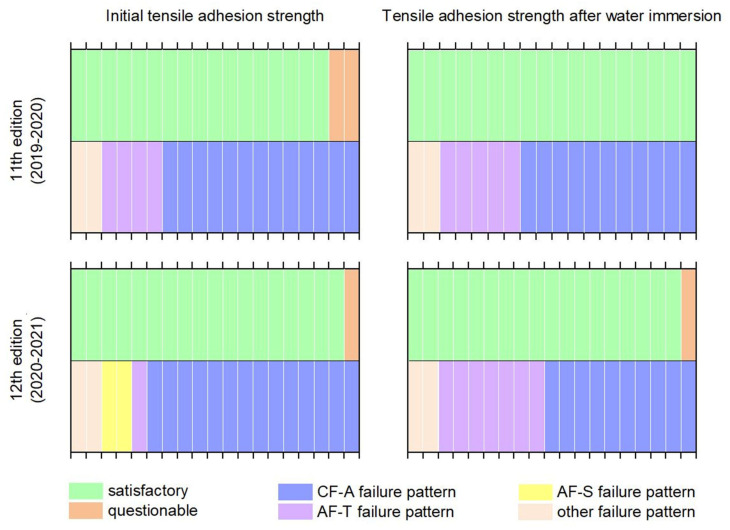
Summary of the results of the CTA measurements in two editions of the ILC.
